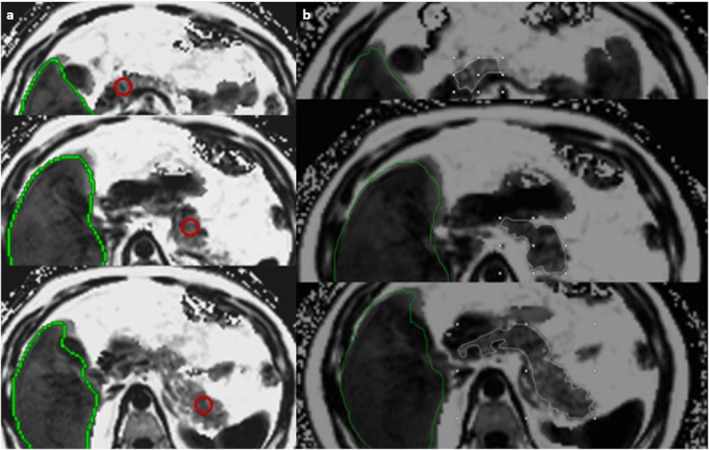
Fat fraction quantification with magnetic resonance imaging‐proton density fat fraction. It is both feasible to quantify pancreatic fat with three regions of interest (ROIs) placed on the pancreatic head, body, and tail to report the average (a) and to measure the entire pancreas using free‐hand ROIs (b).
